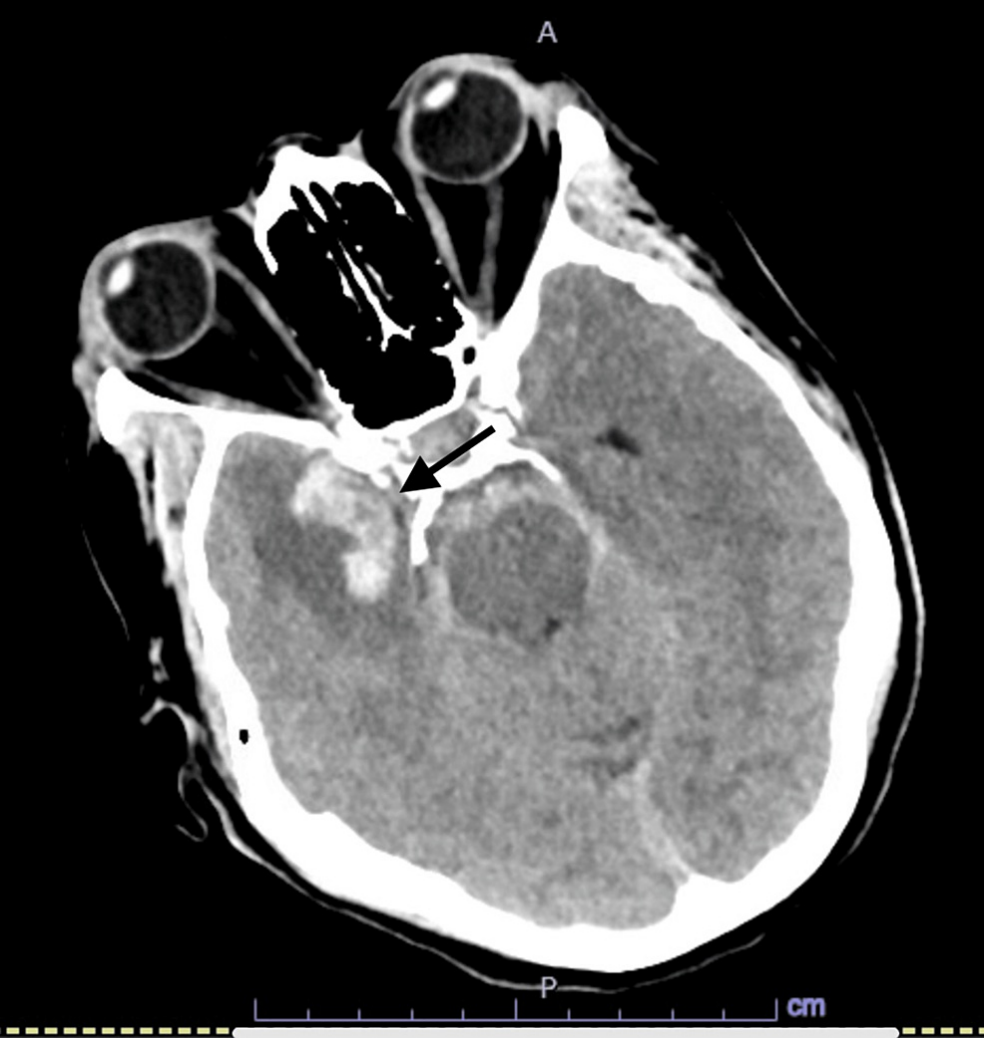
CT Head Computed tomography (CT) head showing evidence of right-sided subarachnoid hemorrhage (arrow).
